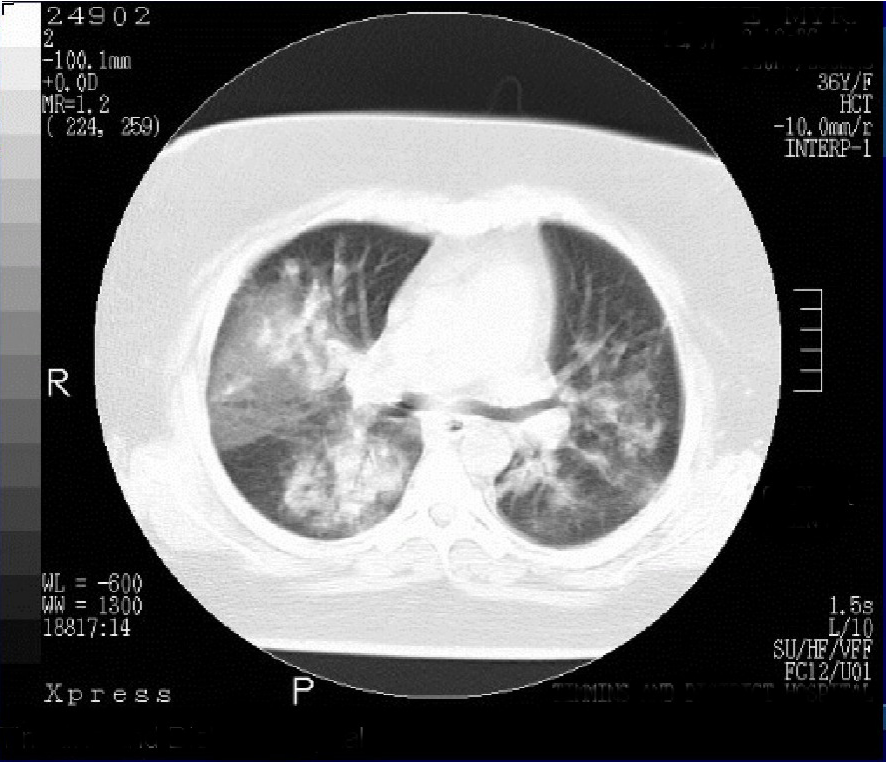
A section of CT scan of chest performed 8 days after hospitalization showing bilateral consolidation of lungs, mainly of lower lung fields.
